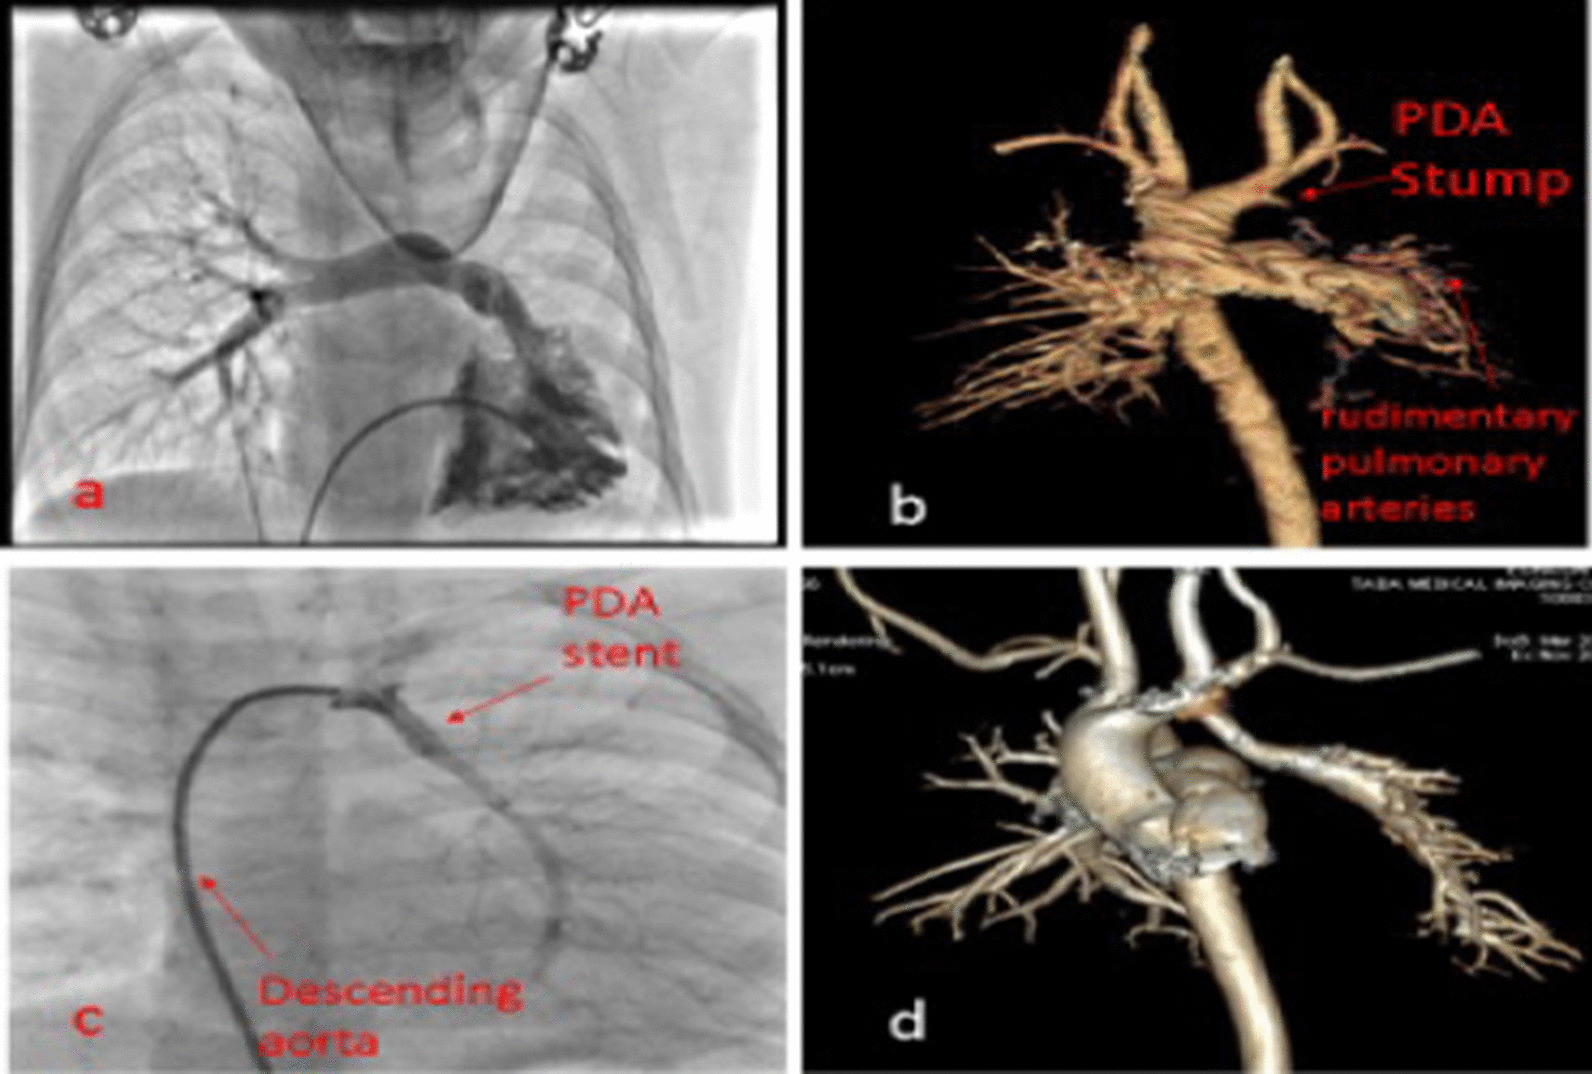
Successful stenting of the ductus arteriosus to the left pulmonary artery in our third patient; a right ventriculography; b CT angiography before the procedure; c stenting of the left pulmonary artery; d CT angiography six months after the procedure
